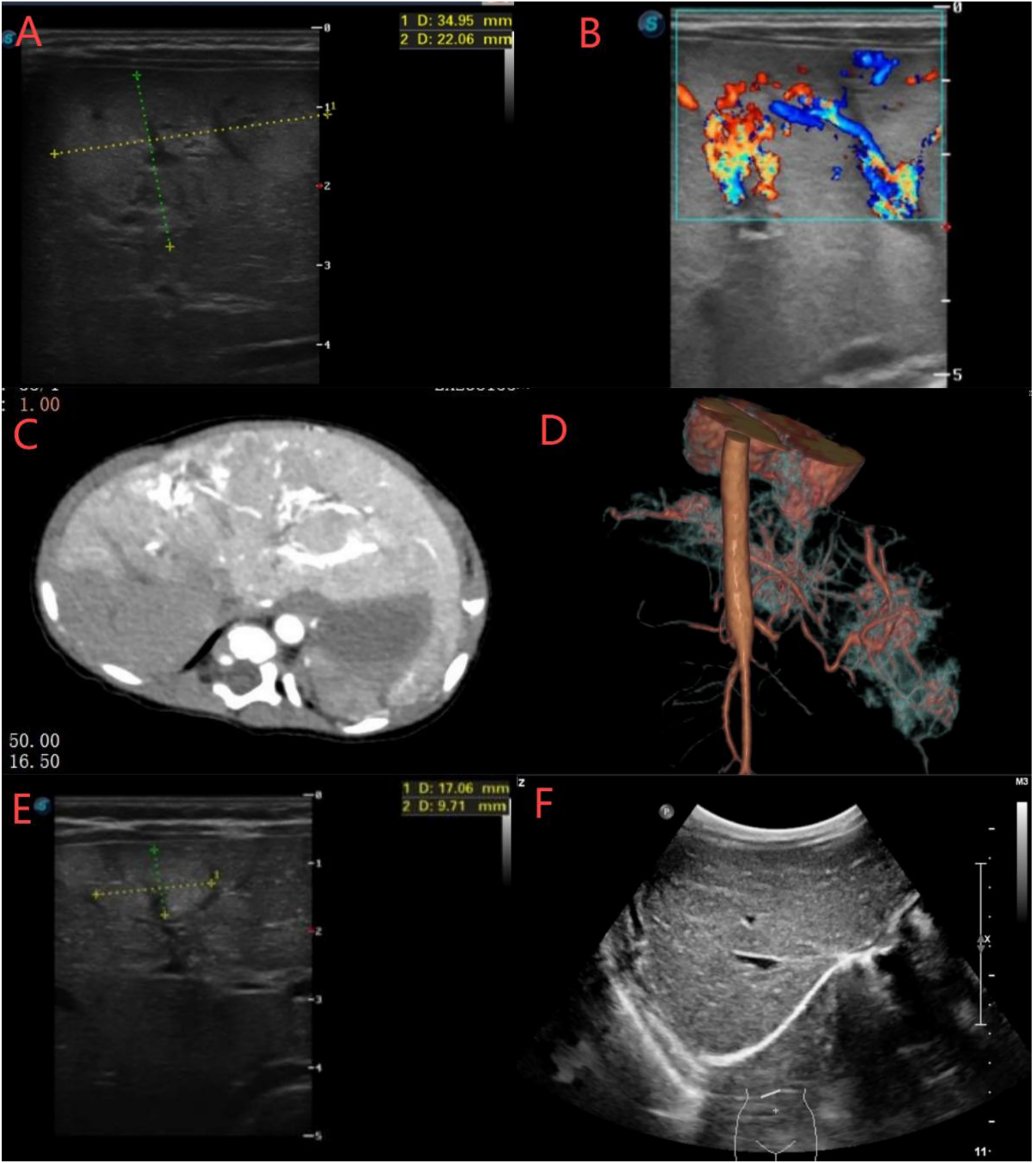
(A,B) Ultrasound showed multiple hyperechoic areas in the left and right hepatic lobes with cavernous echotexture and disordered vascular proliferation. (C,D) CT revealed significant liver enhancement with four large, irregular “snowflake”-like areas containing tortuous and dilated vessels. (E,F) Ultrasound showed that the hepatic hemangiomas gradually decreased in size and completely disappeared at month 18.
